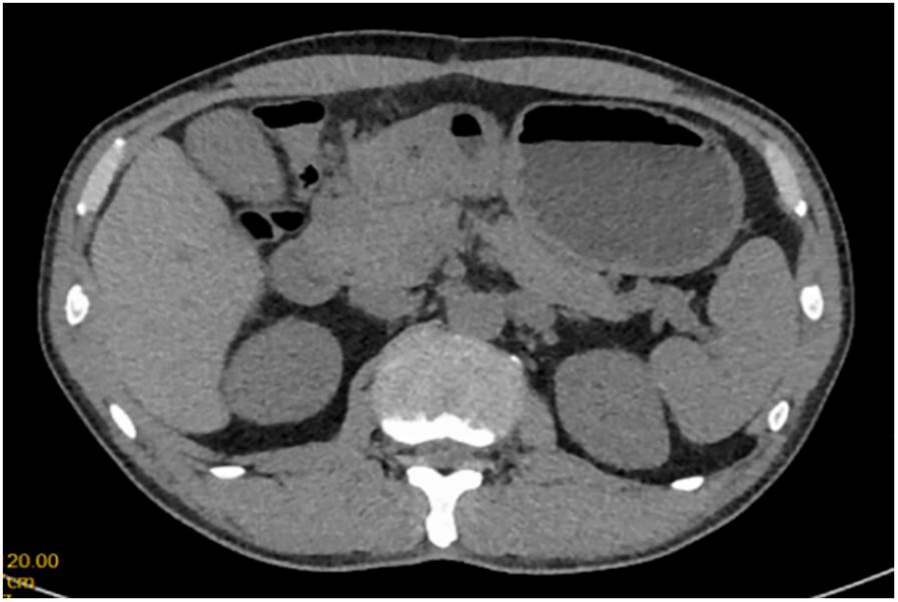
CT scan of duodenal bulb lesion.
